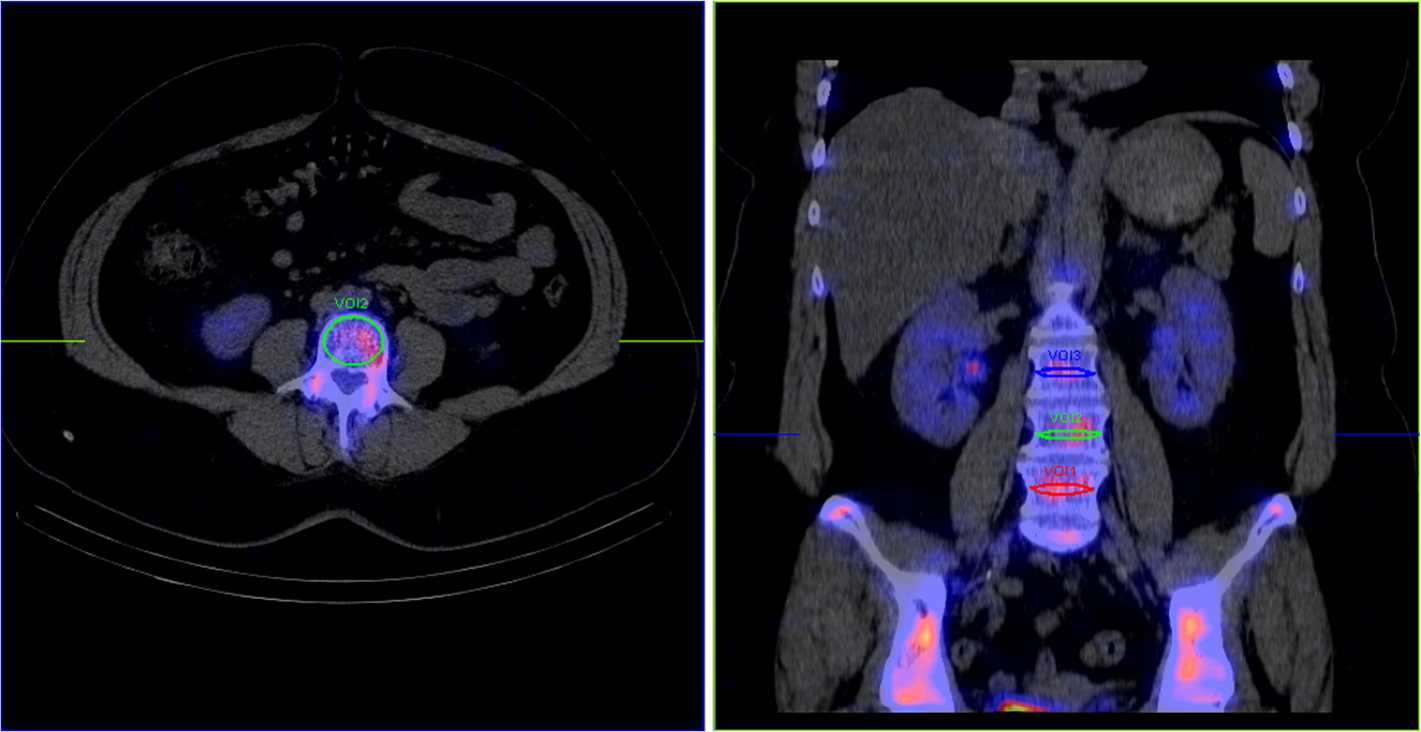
Transverse and coronal images of a patient's SPECT/CT fused data sets including the three lower LV. The ellipses depict the VOIs selected.
